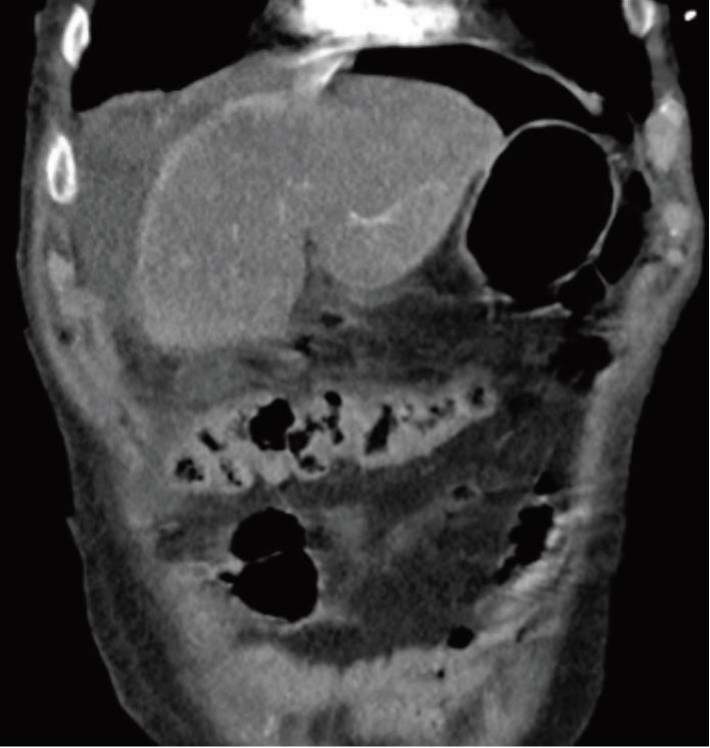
Coronal CT image obtained on arrival demonstrates pneumoperitoneum and intra-abdominal free fluid, consistent with duodenal perforation.
